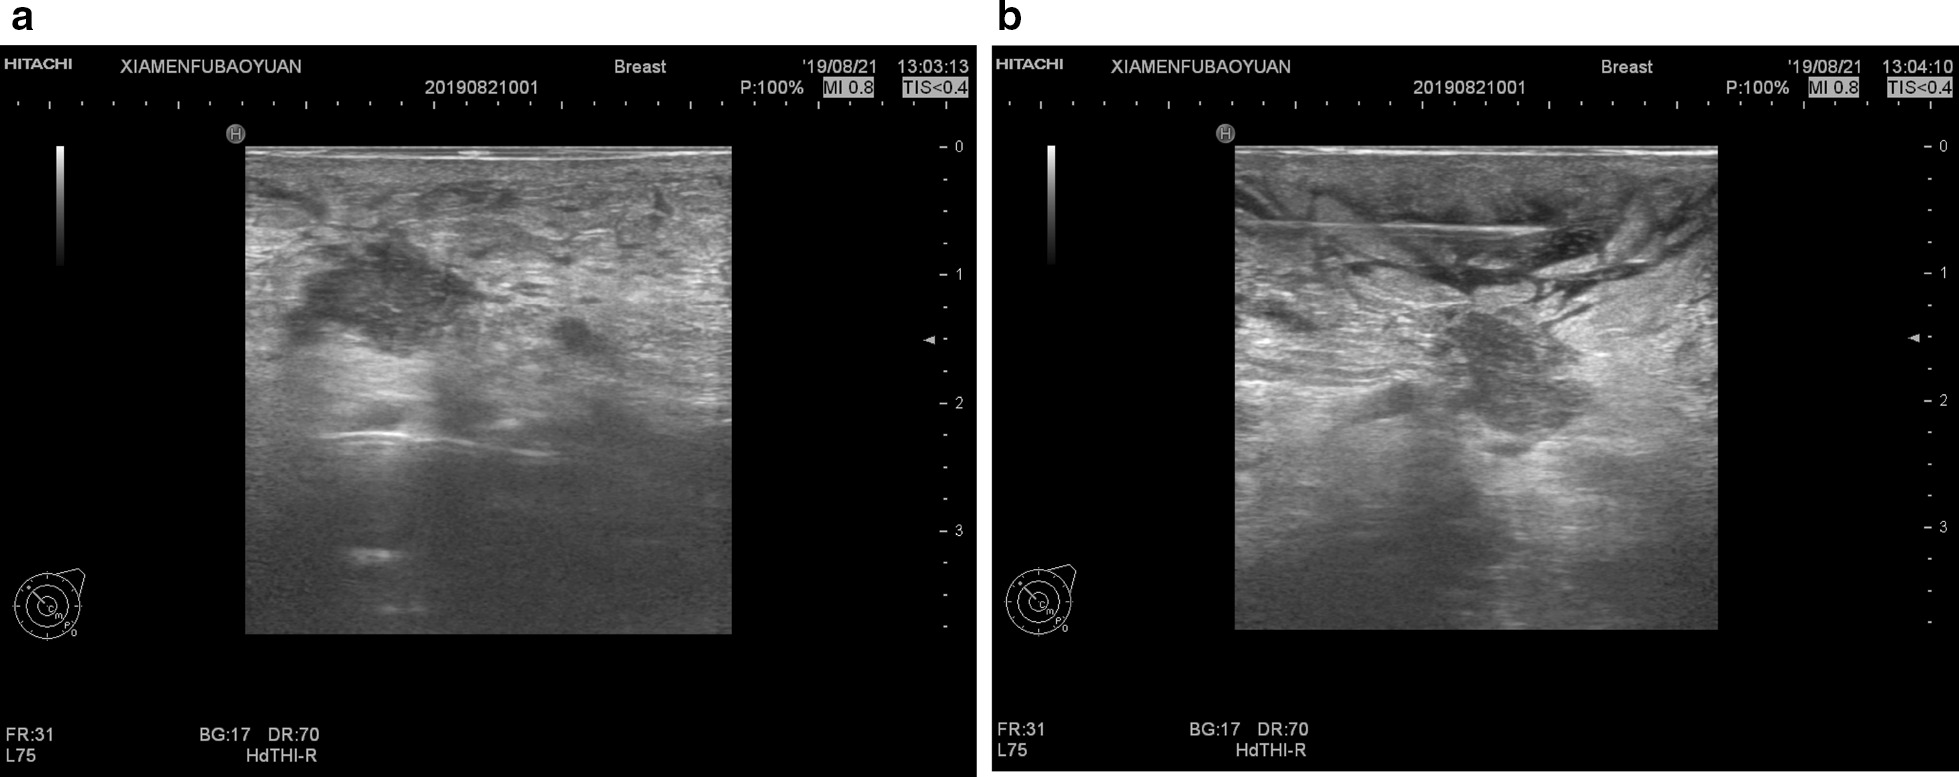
Local infiltration of anesthesia guided by ultrasound. a Anesthesia in the posterior breast space; b Anesthesia in the subcutaneous tissue space
